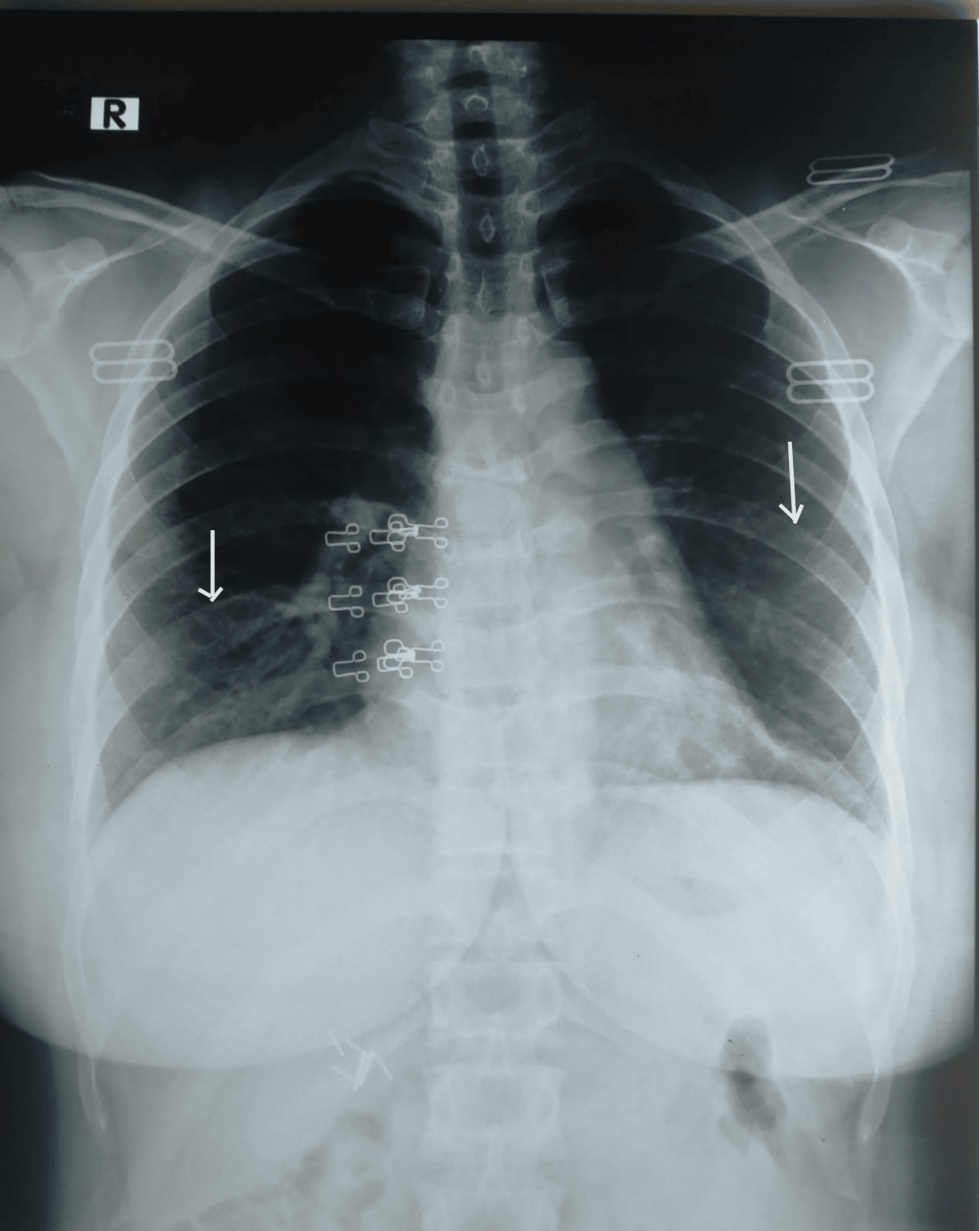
Chest X-ray (Day 1) with arrows showing bilateral mild interstitial infiltrates in the lower zones of the lung.
